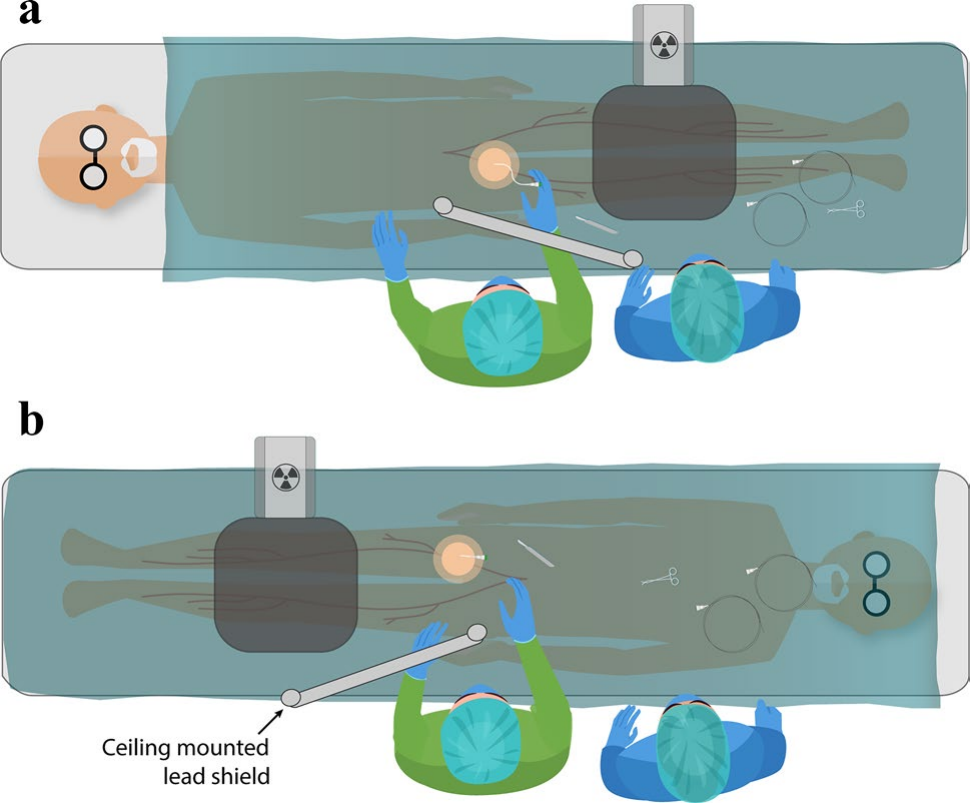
Location of operator and circulator nurse in relation to the x-ray gantry and the ceiling mounted lead shield during; a single leg-normal (SL-N); b single leg-downhill (SL-DH)
